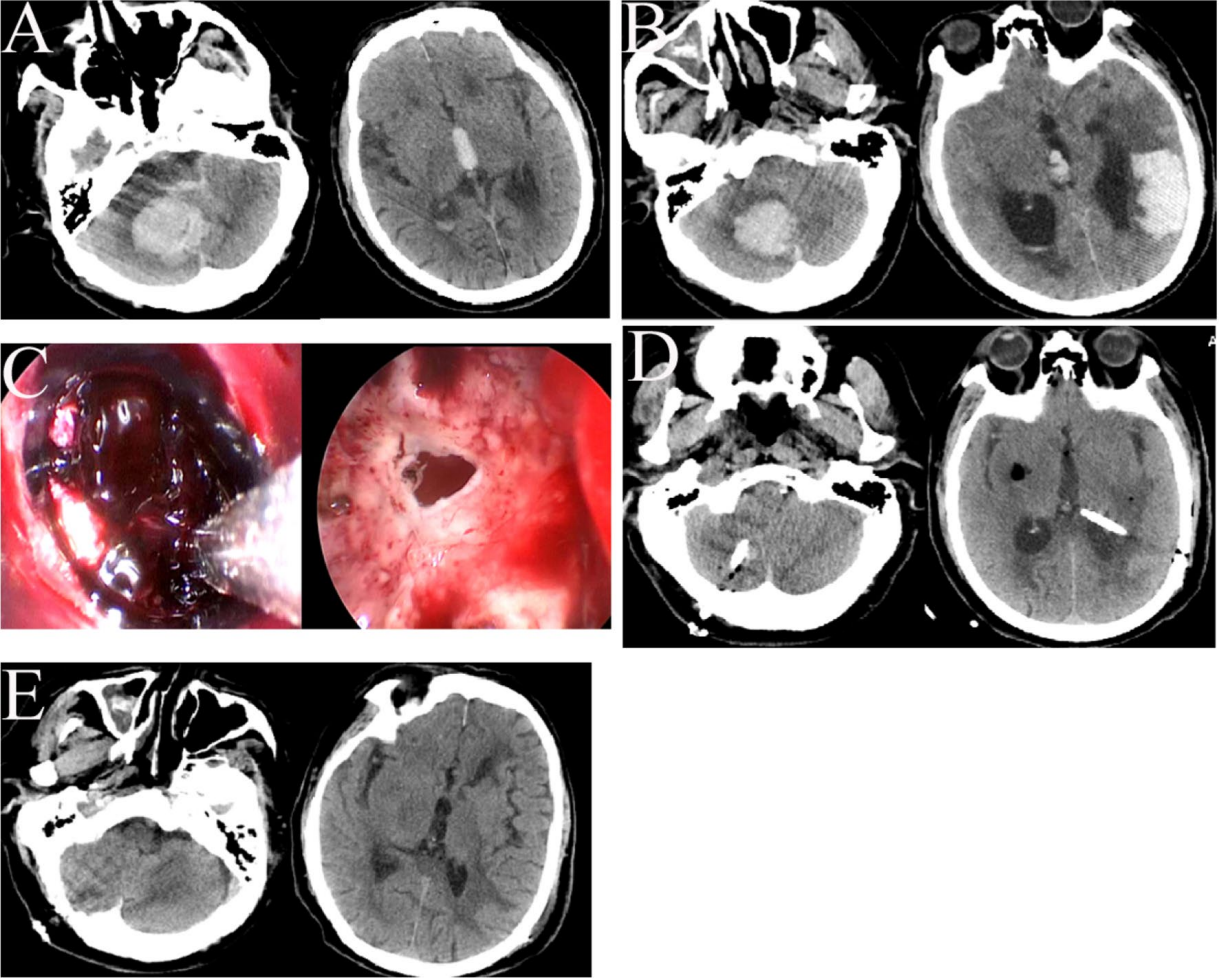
A right cerebellar hemorrhage and a left temporal lobe hematoma were evacuated by transcranial neuroendoscopy. (A) CT showed a hematoma in the right cerebellum at admission. (B) Two days later, a temporal lobe hematoma began to emerge, as well as hydrocephalus. (C) The left temporal lobe and right cerebellum hematomas were removed under neuroendoscopy. (D) CT showed excellent hematoma removal on the day after the operation. (E) CT showed no signs of hematoma before discharge.
